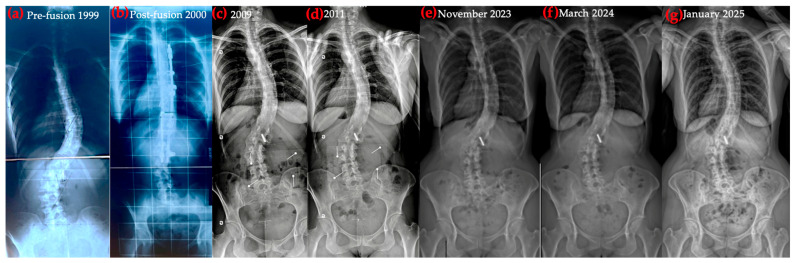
Coronal X-ray progression over time: (a) pre-surgery; (b) post-surgery; (c,d) coronal postural deterioration; (e) coronal postural improvement, after 1 year Schroth + brace; (f) coronal postural improvement, after 17 months Schroth + brace; (g) coronal postural improvement, after 28 months Schroth + brace.
